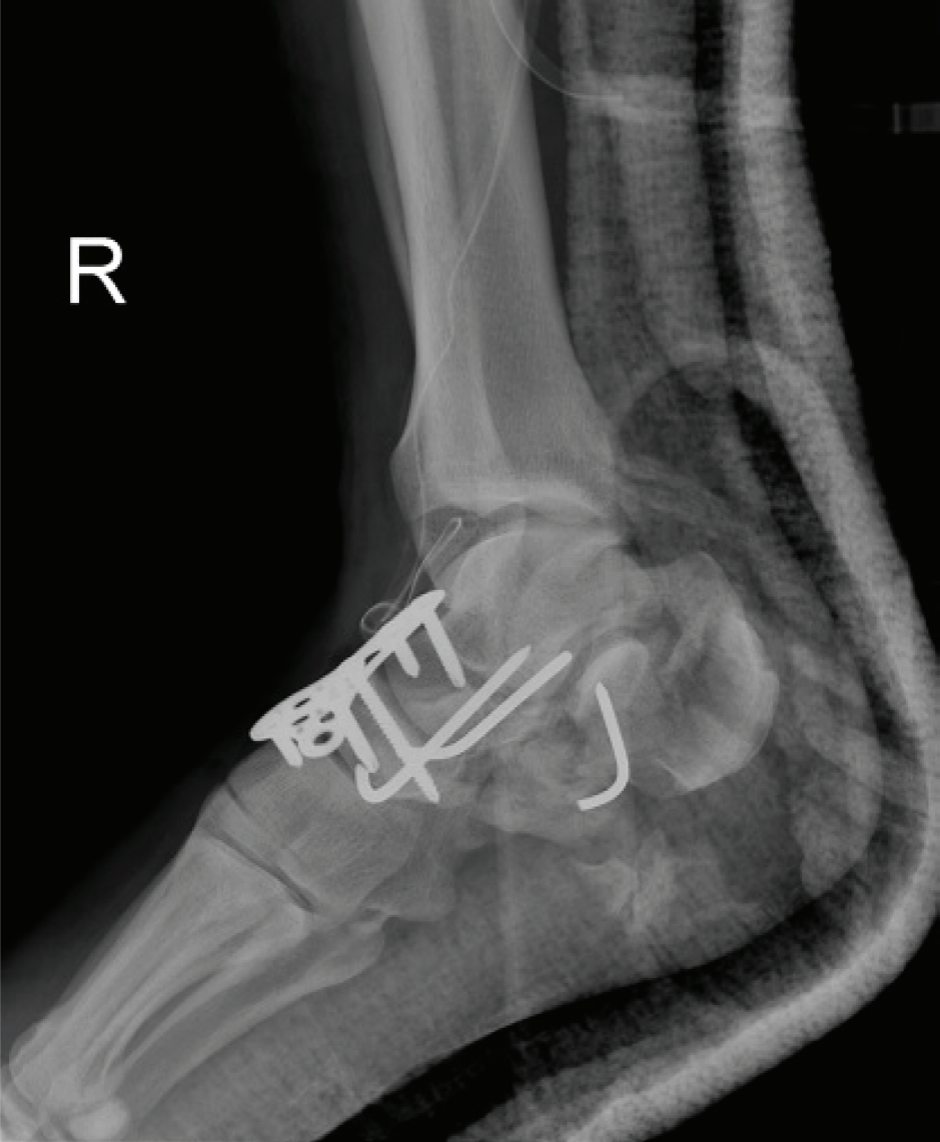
Lateral radiograph after the first surgery
